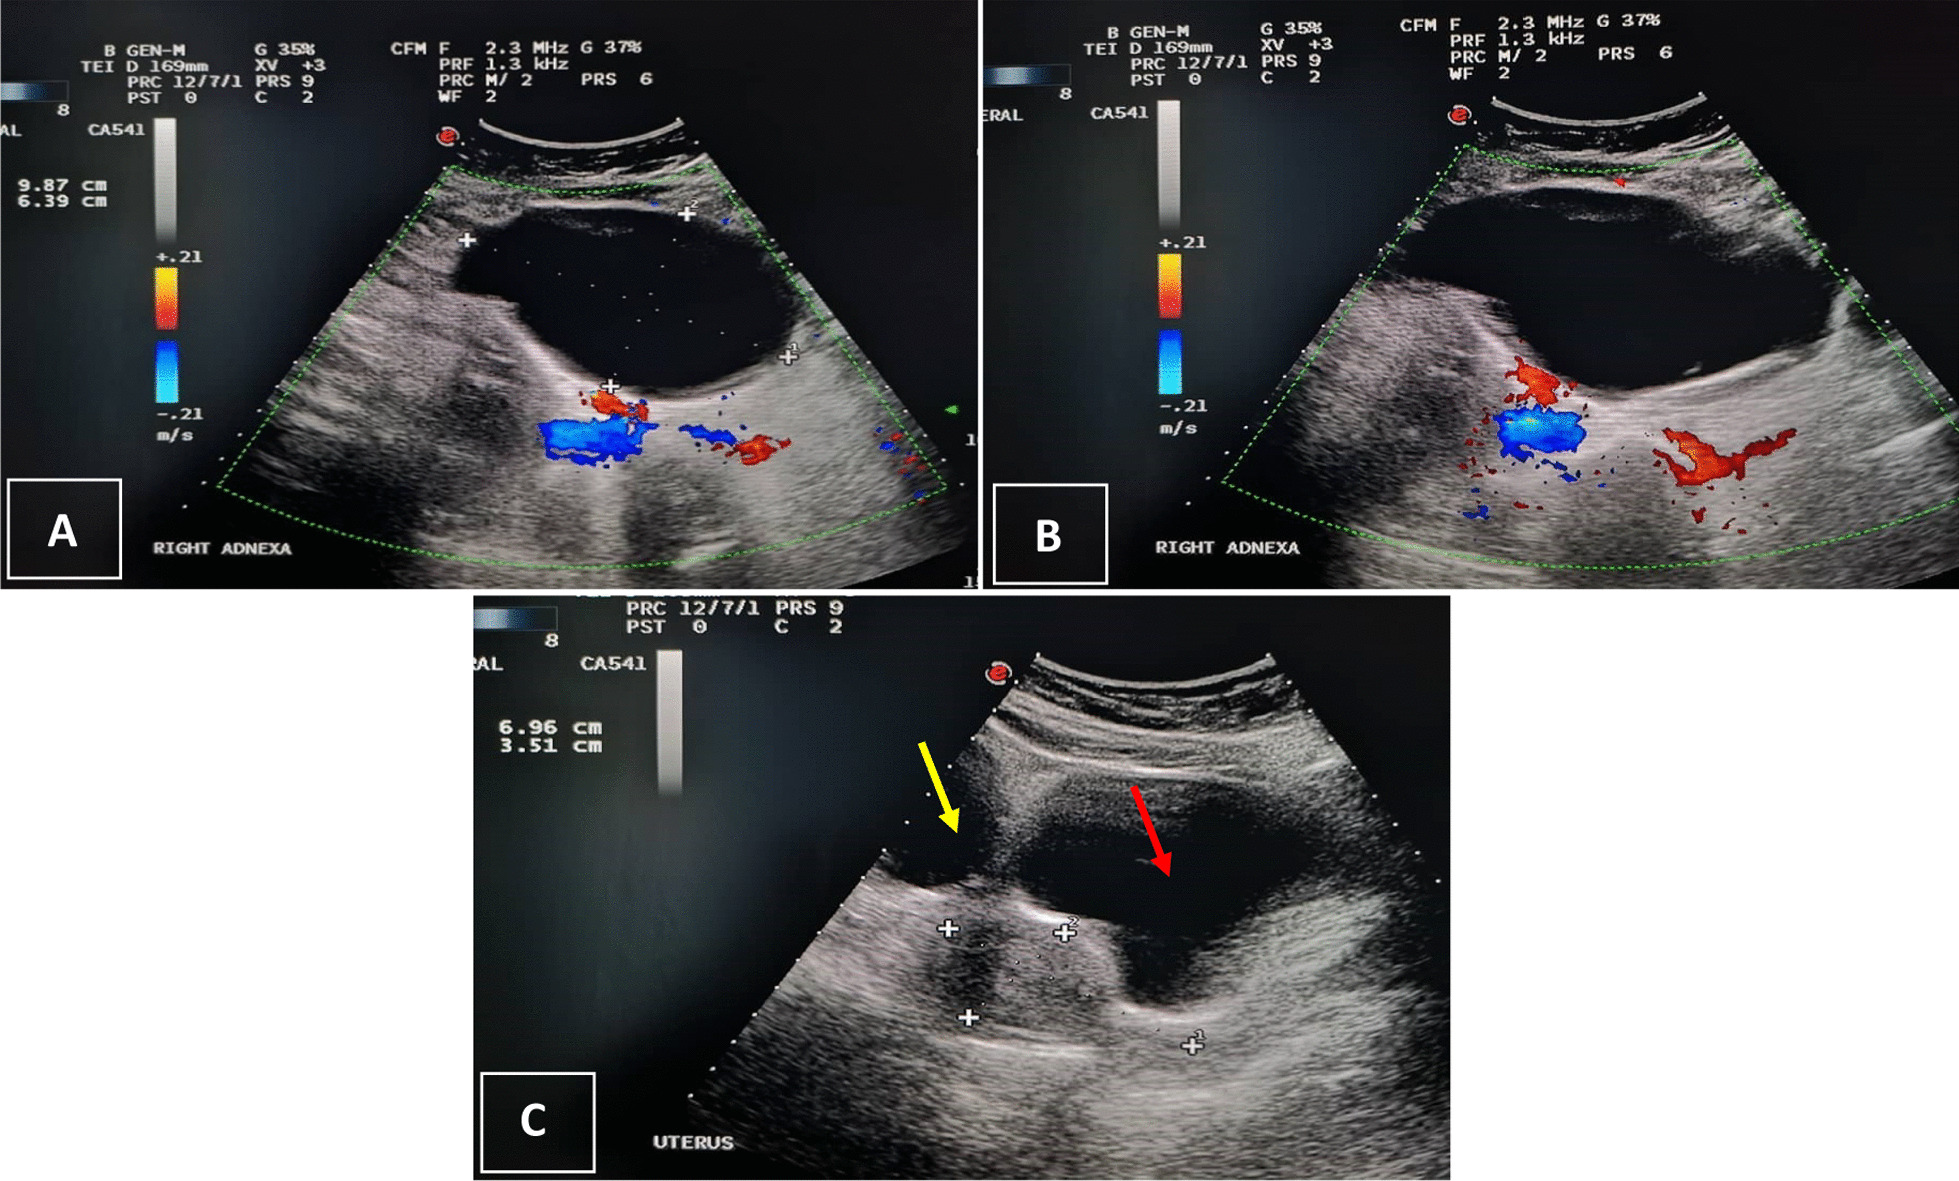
Pelvic ultrasound A and B showing median and right paramedian pelviabdominal cystic, thin-walled lesion of a pear to ovoid shape with few very thin septa and some hyperechoic material in its dependent part. The lesion shows no calcifications or vascularity internally in its wall. C Sagittal/vertical midline view of the pelvis showing the uterus (++++) and the urinary bladder (red arrow) just anterior to it, and showing part of the cystic lesion (yellow arrow) located anterosuperior to the uterus
